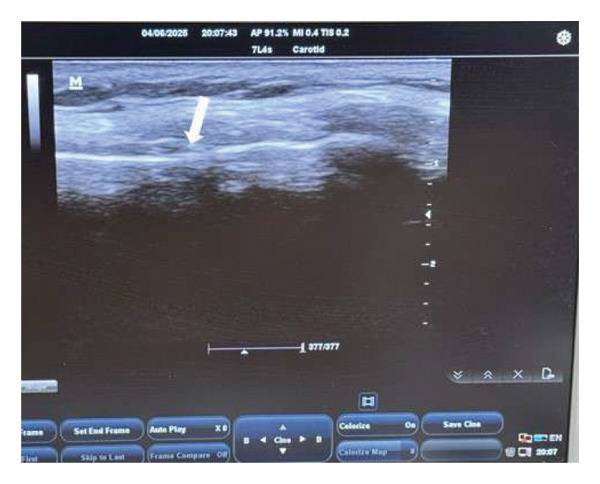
Recurrent laryngeal nerve block. An ultrasound image shows the anatomical landmarks for the injection site. The arrow shows the cricothyroid membrane, which is the injection site.
